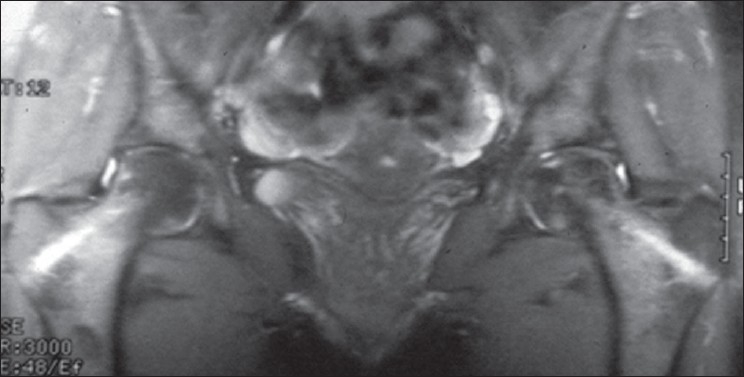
Immediate postoperative MRI (T2 weighted images) after core decompression and autogenous bone marrow grafting showing the channel and changes of signal in the superior part of the femur
